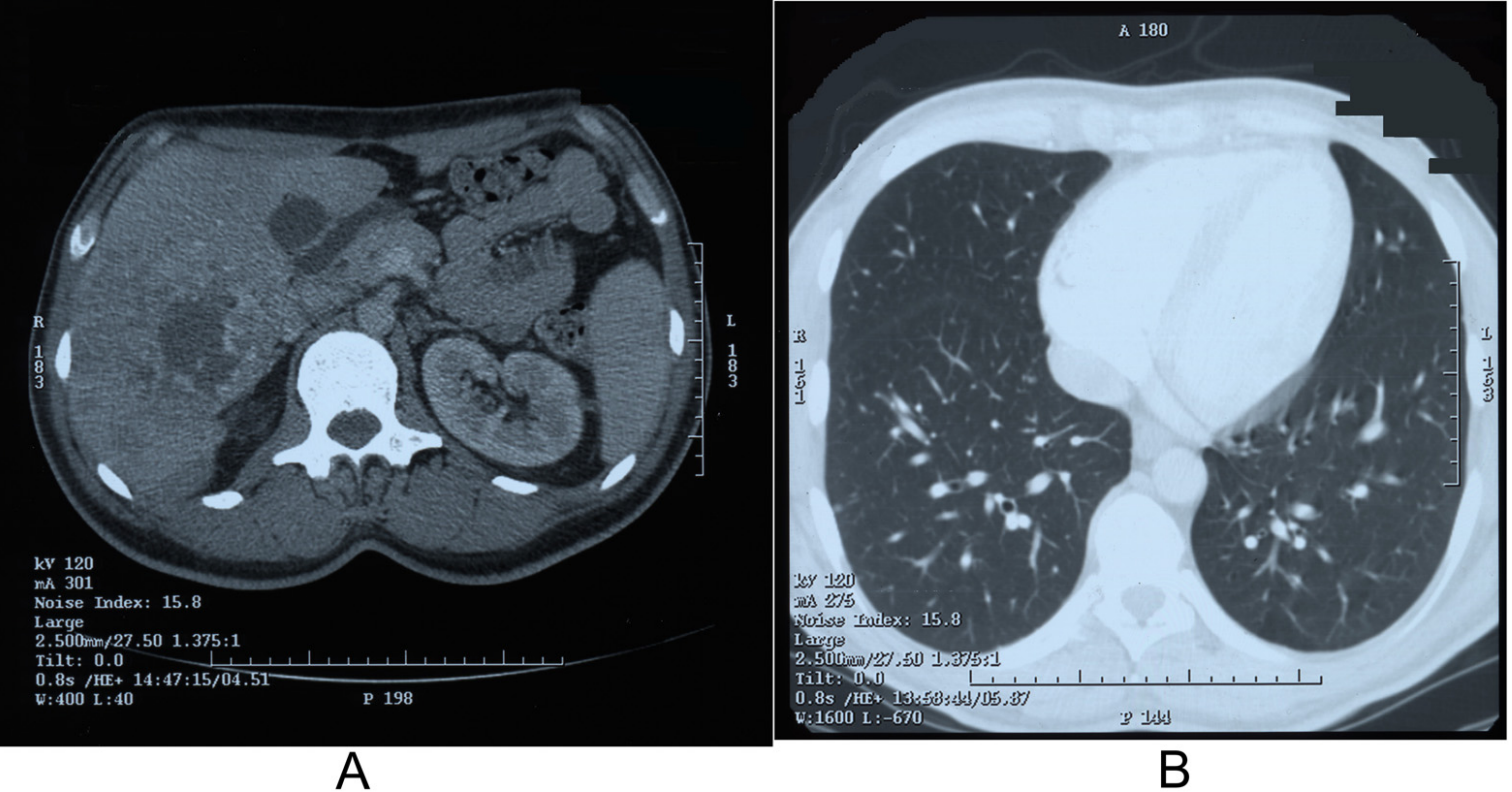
Computed tomography, A – multiple focal changes in liver are visible, B – multiple metastases to lungs are visible.
